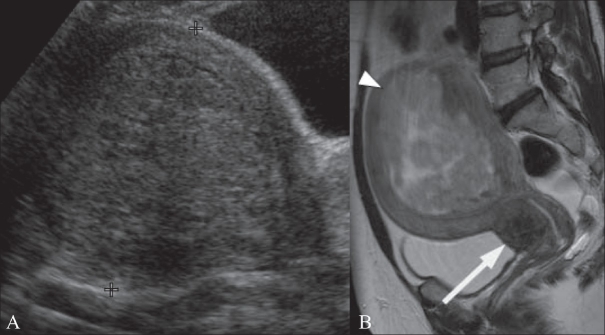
A 49-year-old woman with a history of menorrhagia. Transabdominal (TA) USG image (A) shows a bulky uterus showing a 10-cm submucosal fibroid (between cursors). Sagittal T2W MRI image (B) in the same patient shows that the submucosal fibroid (arrowhead) is heterogeneous indicating degeneration. There is also a 2.5-cm cervical fibroid (arrow)
